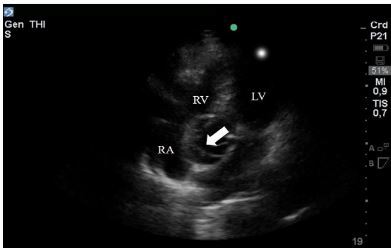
Transthoracic echocardiography apical five-chamber view of a patient in post-cardiac arrest with a dissection flap (white arrow) visualized in the ascending aorta. *LV*, left ventricle; *RV*, right ventricle; *RA*, right atrium.
